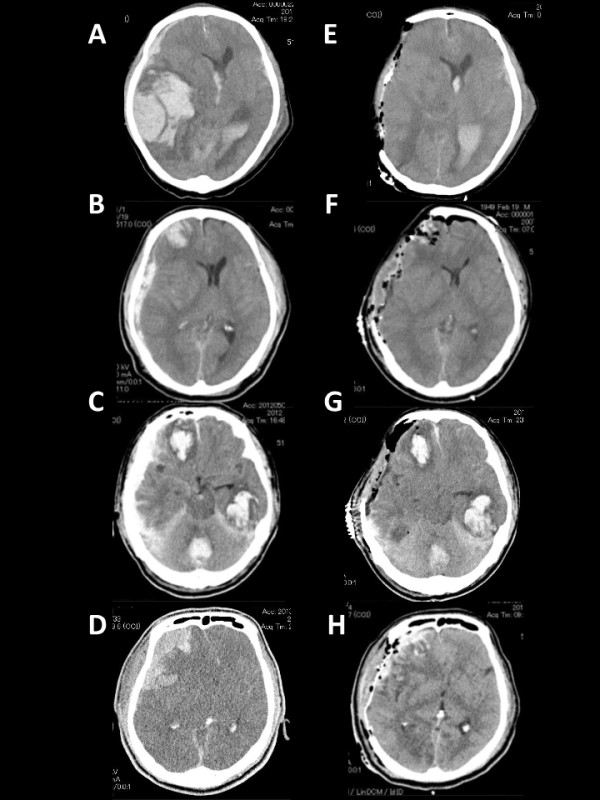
Pre- (A, B, C, D) and postoperative (E, F, G, H) CT scans of four right ASDH patients with contusion, none of whom sustained intractable brain swelling. A, E: a 57-year-old man with GCS score of 5; B, F: a 58-year-old man with GCS score of 3; C, G: a 79-year-old man with GCS score of 5; D, H: a 64-year-old man with GCS score of 6. All postoperative CT scans were obtained within 24 h of surgery.
